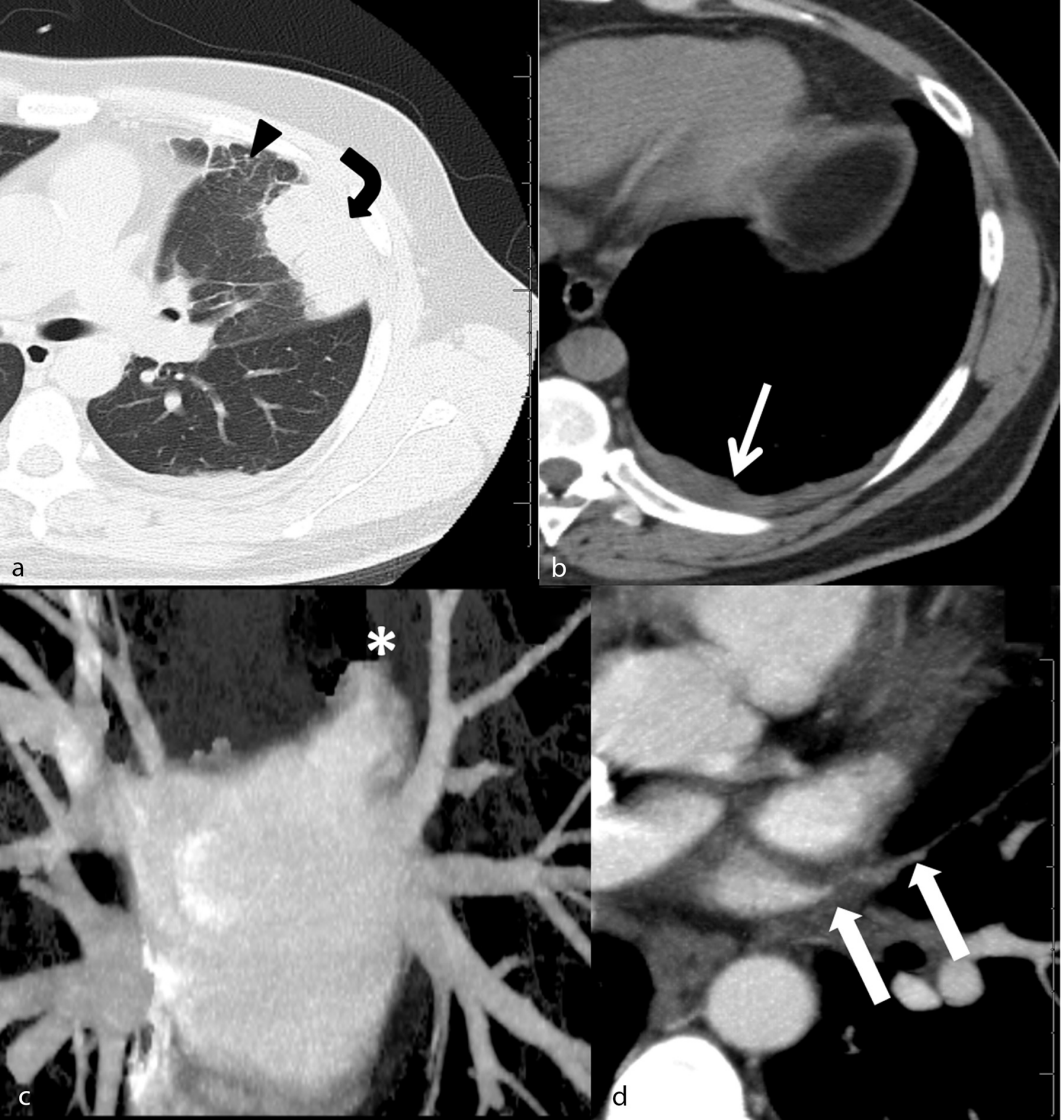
(a and b) Non-enhanced chest CT scan: lung window (a) shows pleural-based parenchymal consolidation (curved arrow) associated with adjacent subtle ground glass attenuation and interlobular septal thickening (arrow head). Mediastinal window (b) shows left-sided minimal pleural effusion (thin arrow). These pulmonary findings are related to total LSPVO revealed by (c) 3D reconstruction of left atrium (asterisk), note other pulmonary veins patency. (d) Contrast-enhanced CT scan axial section depicts total occlusion of LSPV.
